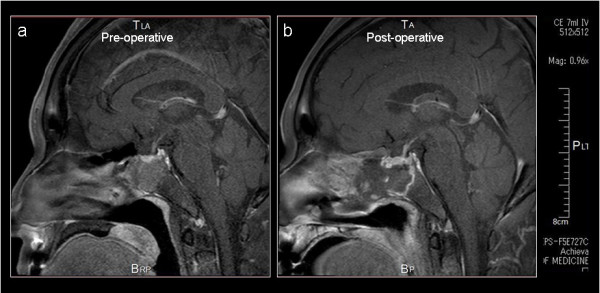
Brain MRI. MRI of pre- (a) and post-operative pituitary tumor (b).
